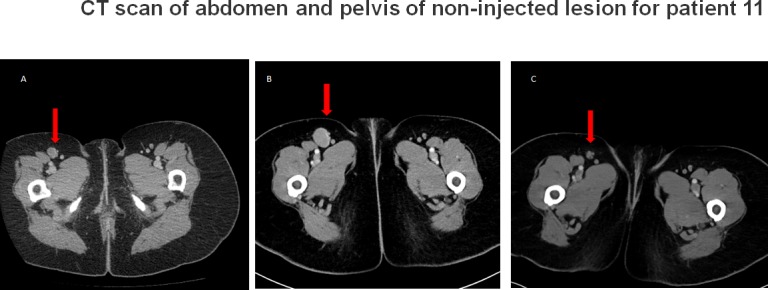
CT scan of abdomen and pelvis of non-injected lesion for patient 11 (shown by the arrow) at A. screening B. 1 months post last injection and C. 4 months post last injection. Initial progression followed by regression was observed in (B) and (C), respectively. This figure reflects both pseudo-progression and abscopal response.
